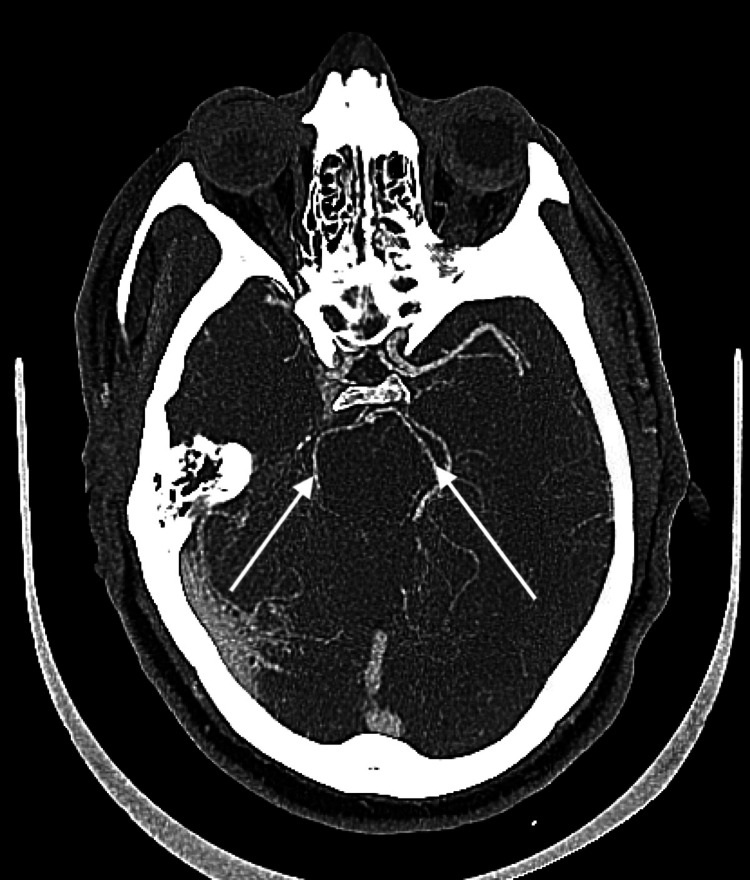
Computed tomography angiogram of the head, axial view: bilateral posterior cerebral arteries appear patent.
